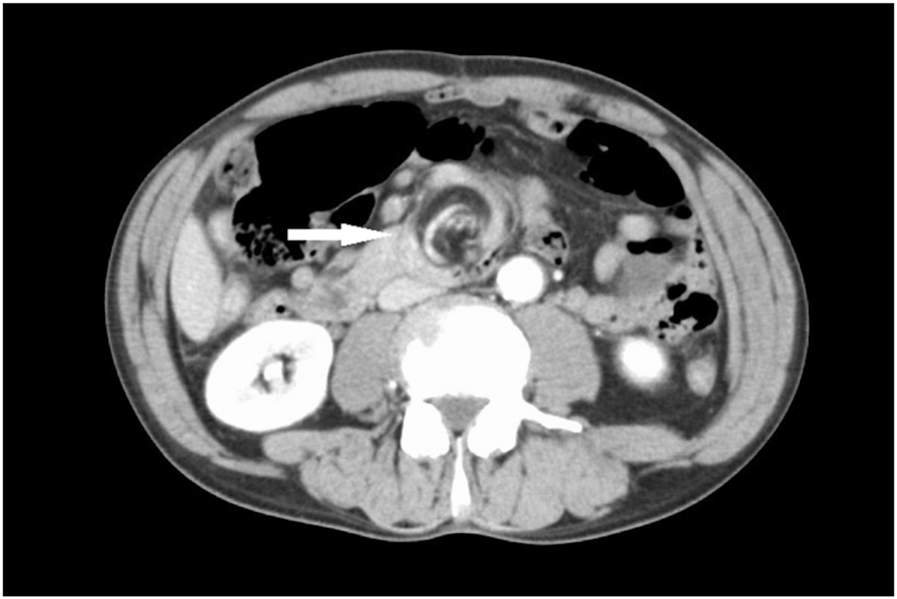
Enhanced abdominal CT shows the whirl sign (arrow) involving the superior mesenteric artery as the center
